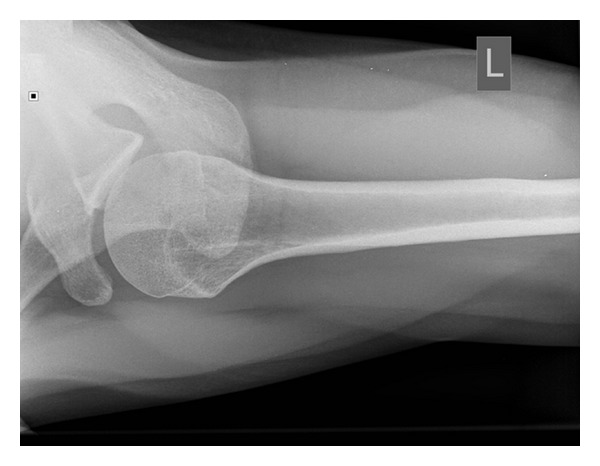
Axillary view radiograph taken 3 weeks after initial injury.
